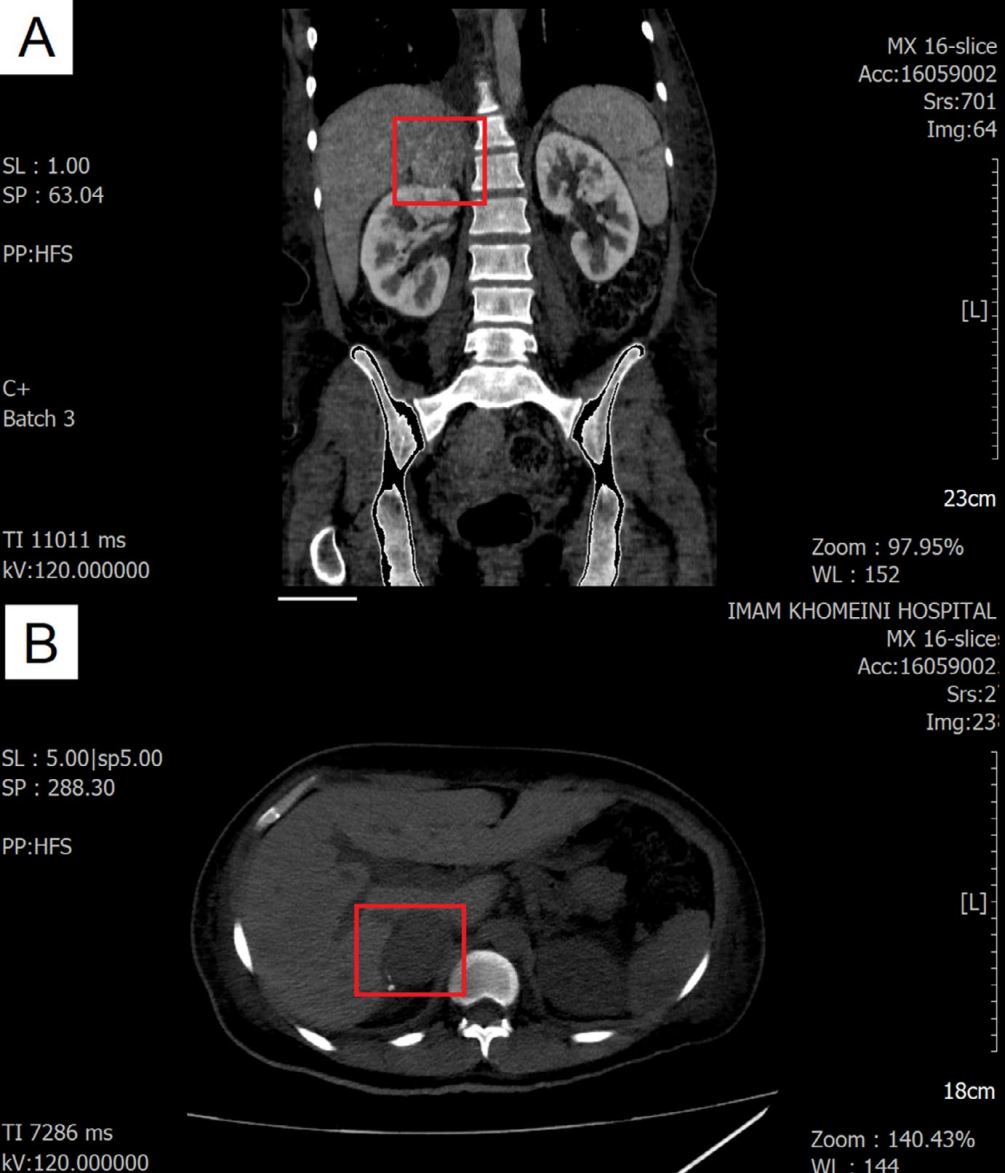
Right adrenal mass. The red square indicates the tumor area. Non‐contrast CT scan showing a Hounsfield unit of 36. After 2 min, the relative and absolute washout rates are 26% and 47%, respectively.
